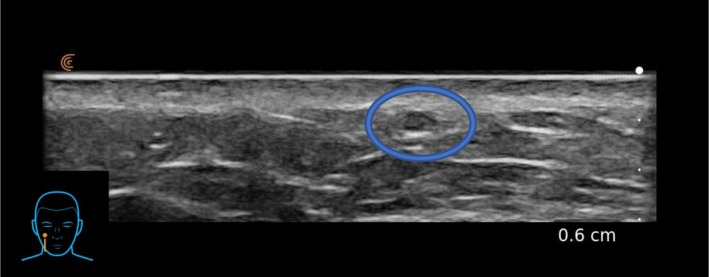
Subject's superficial HA bleb as seen on ultrasound imeediately after intradermal injection of CPM‐HA20G.
